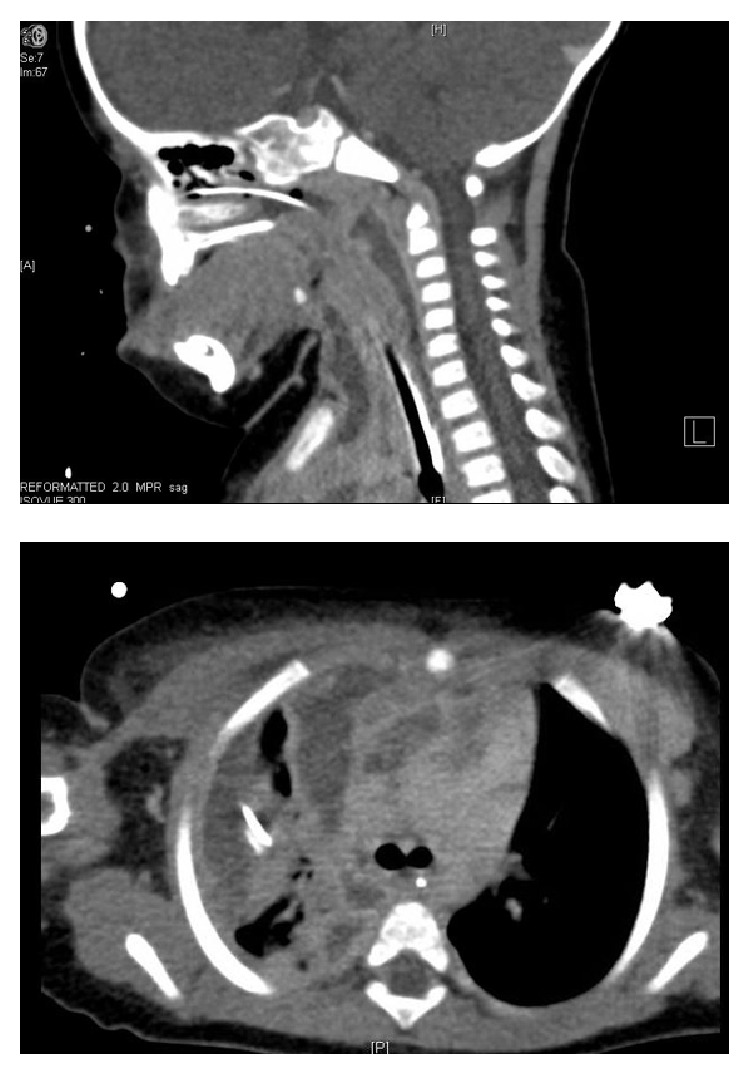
Sagittal and transverse CT images.
